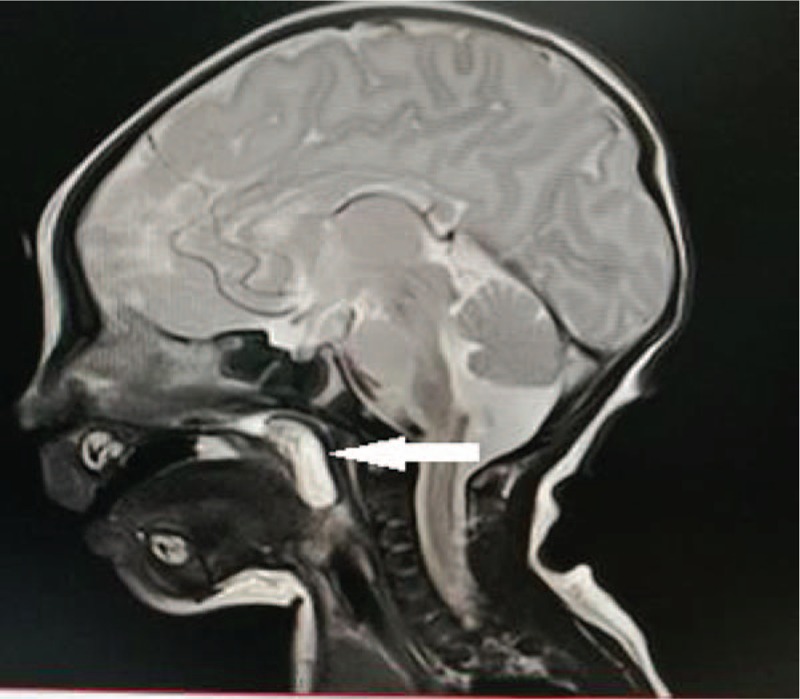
Clear boundaries of T2-weighted MRI image prompted adipose-derived mass (patient #1).
